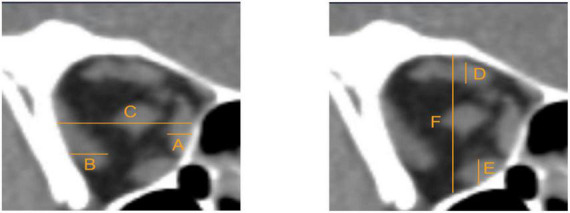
Measurements were taken at the coronal CT scan halfway between the posterior globe and orbital apex. (A) Medial rectus muscle. (B) Lateral rectus muscle. (C) Orbital width. (D) Superior rectus/levator complex. (E) Inferior rectus muscle. (F) Orbital height. The horizontal muscle index = (A+B)/C. The vertical muscle index = (D+E)/F. The larger one of these two indexes was taken as final muscle index.
